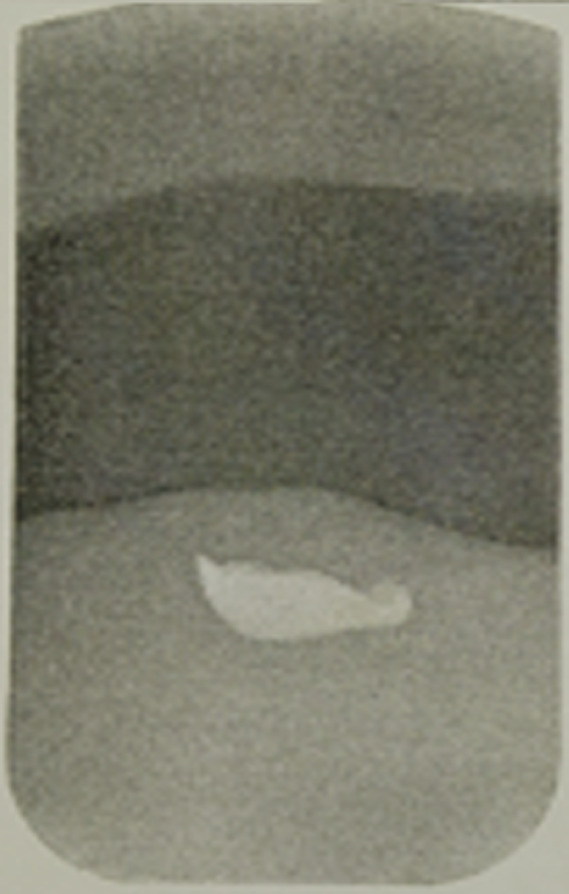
radiograph of the lower lip showing the radiopaque tooth fragment
